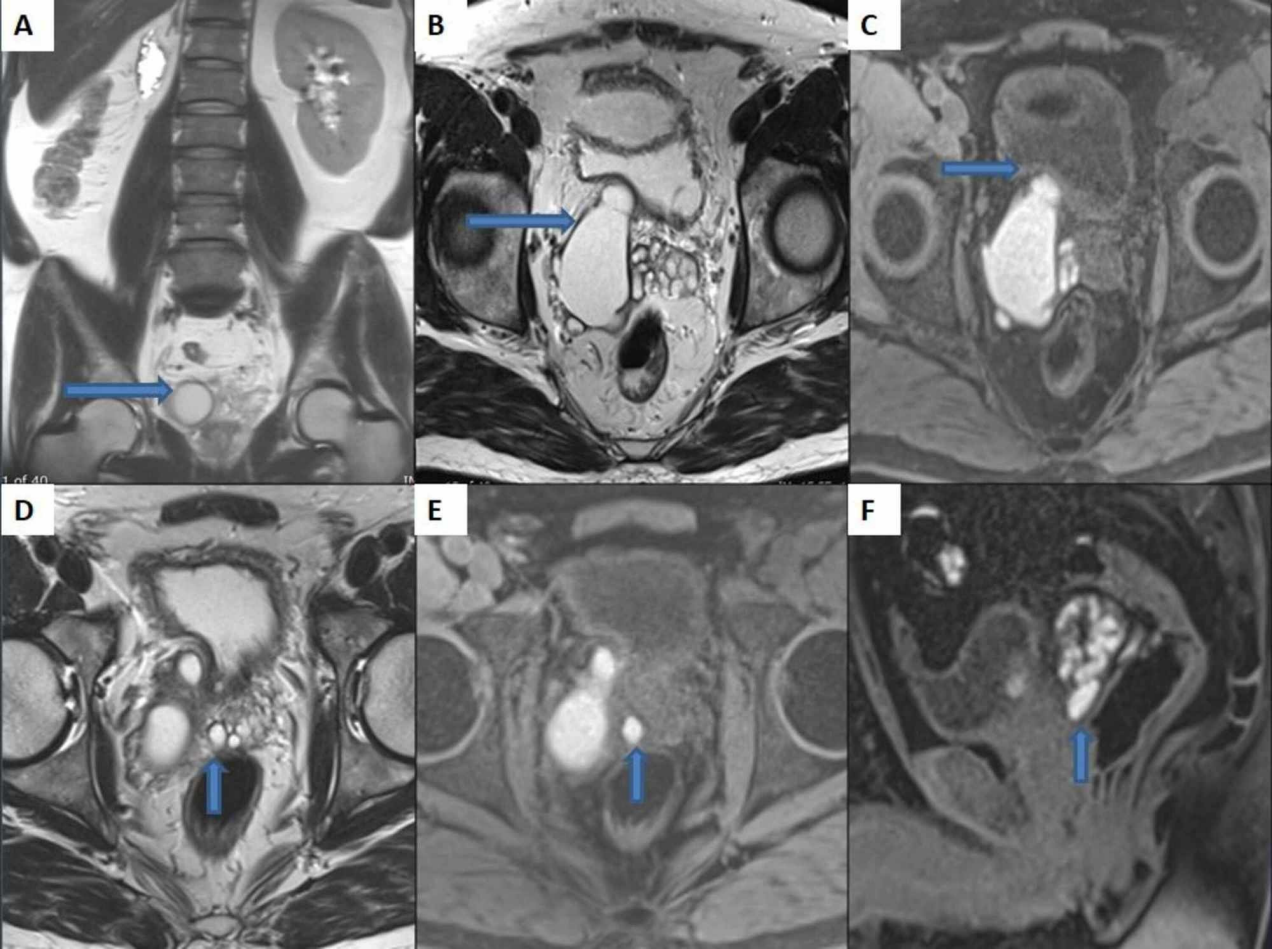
MRI of the pelvis Wide field of view coronal T2-weighted image (A) showing absent right kidney and a structure in the right hemipelvis with high T2 signal intensity (cystic). Axial T2 (B) and axial T1 fat-sat (C) images show cystic dilatation of right seminal vesicle (6 x 2.5 cm in anteroposterior and transverse dimensions, respectively) with T1-hyperintense hemorrhagic or proteinaceous content, which is indenting the right posterolateral urinary bladder wall. Axial T2 (D), axial T1 fat-sat (E), and sagittal T1 fat-sat (F) images show dilated right ejaculatory duct (especially the distal segment which measures 8 mm in diameter) along the right posterolateral aspect of the prostate. The contents of this cystic dilatation are similar to the dilated right seminal vesicle.
